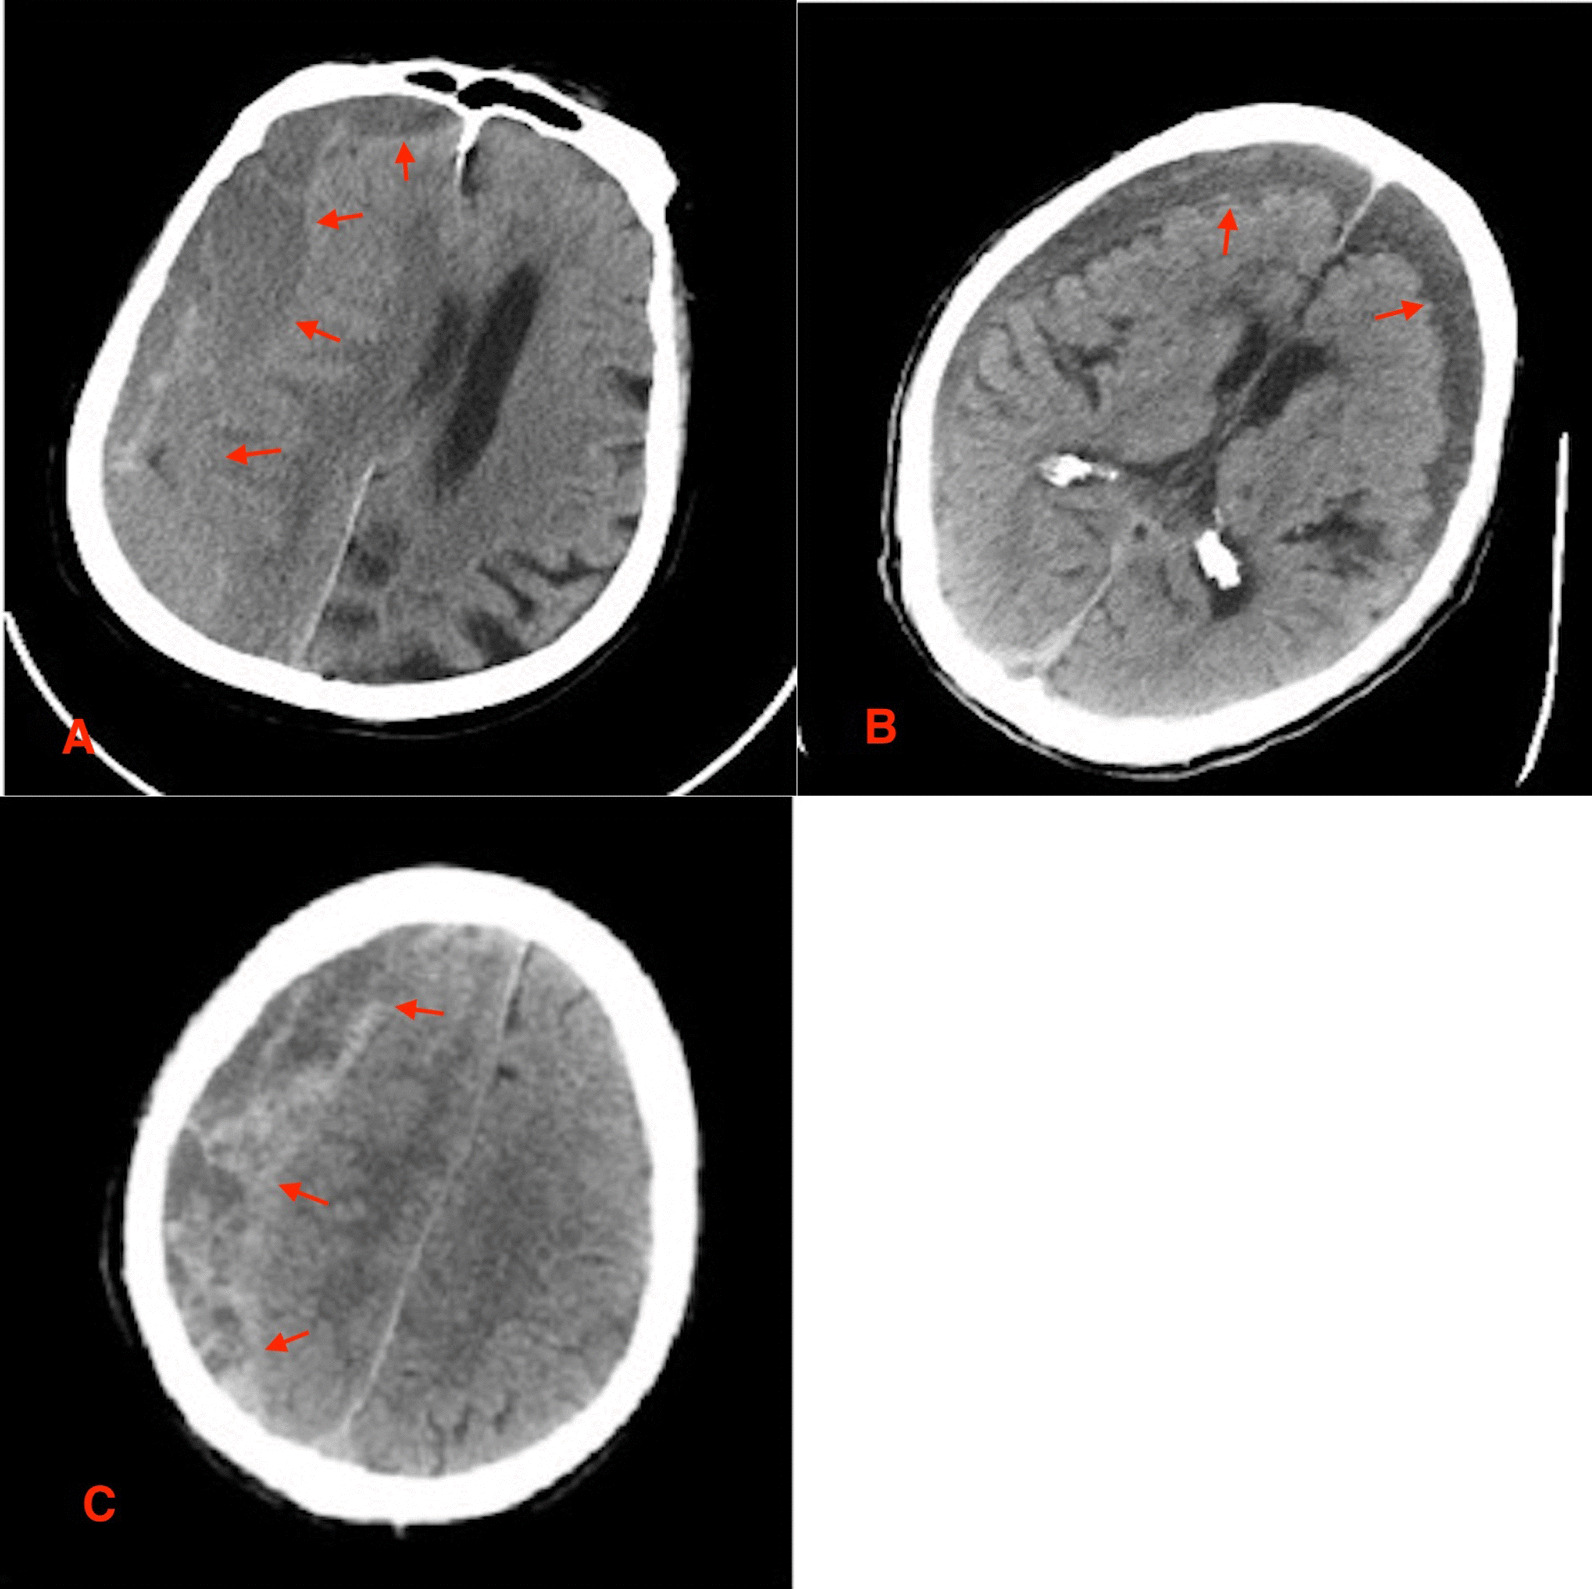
CT characteristic of patients: Hematoma cavity separation, new neomembrane and mixed density hematoma in CT. A, B hematoma cavity separation. C Mixed density hematoma. *Red arrow*  boundaries of the abnormality
